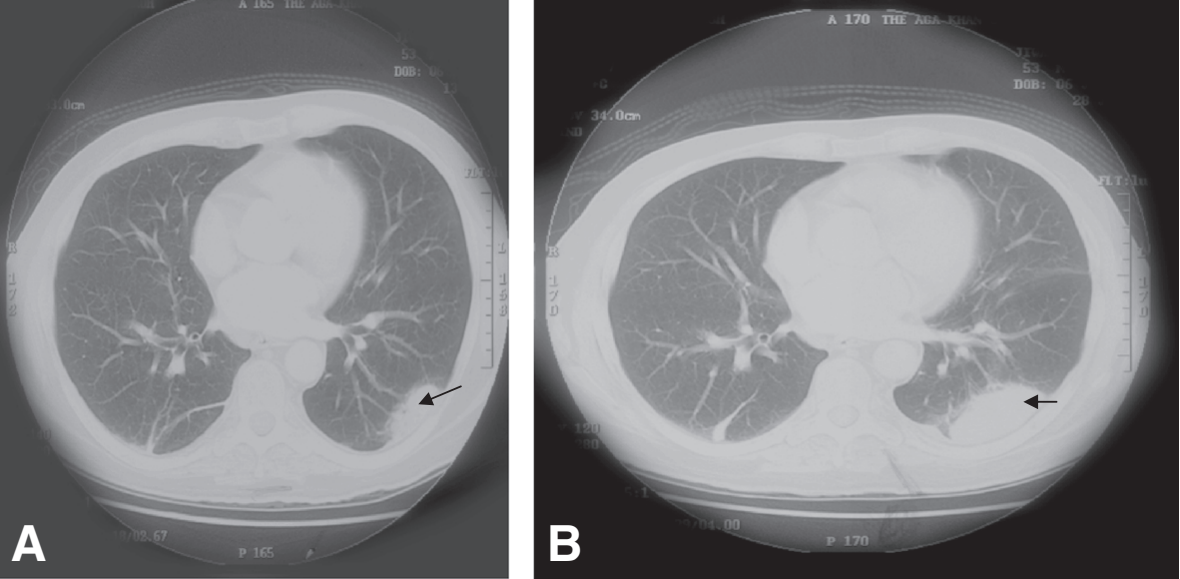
CT Chest before treatment for Nocardia (Case 1). (A) Cavitatory lesion (3.6×2.5×1.8 cm) in left lower lobe lung with adjacent alveolitis. (B) Soft tissue density mass left lower lobe (5.2×3.5×2.8 cm)-near doubling of lesion within span of 14 days.
